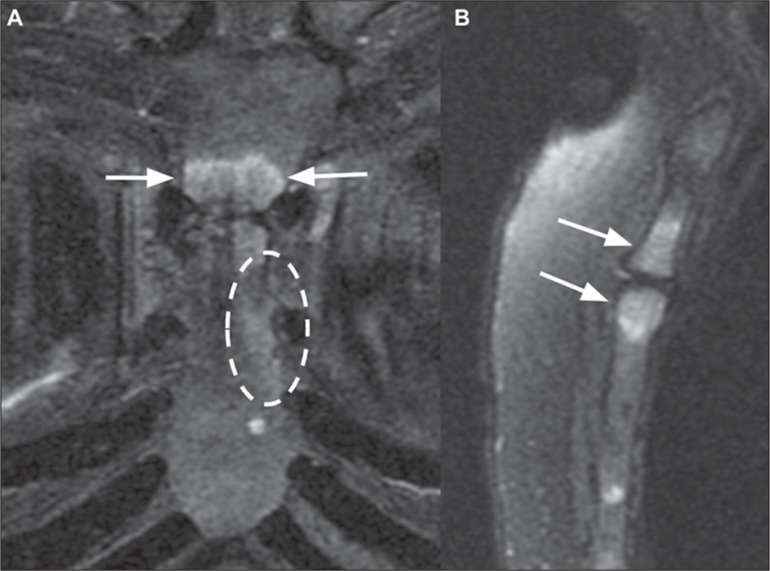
Tietze syndrome. A: Coronal T2-weighted MRI with fat suppression, showing
